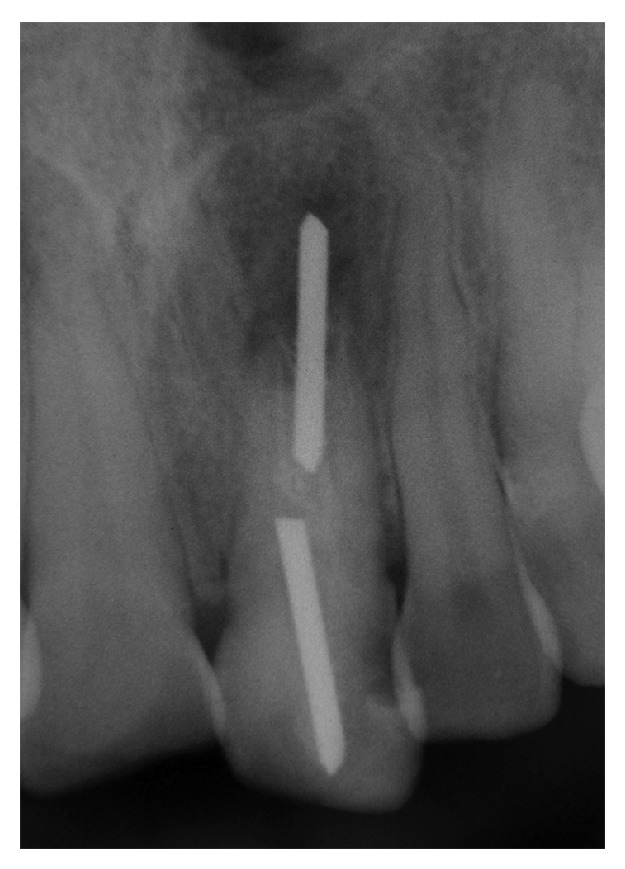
The initial radiograph showing the radiopaque body invading the periapical tissues.
